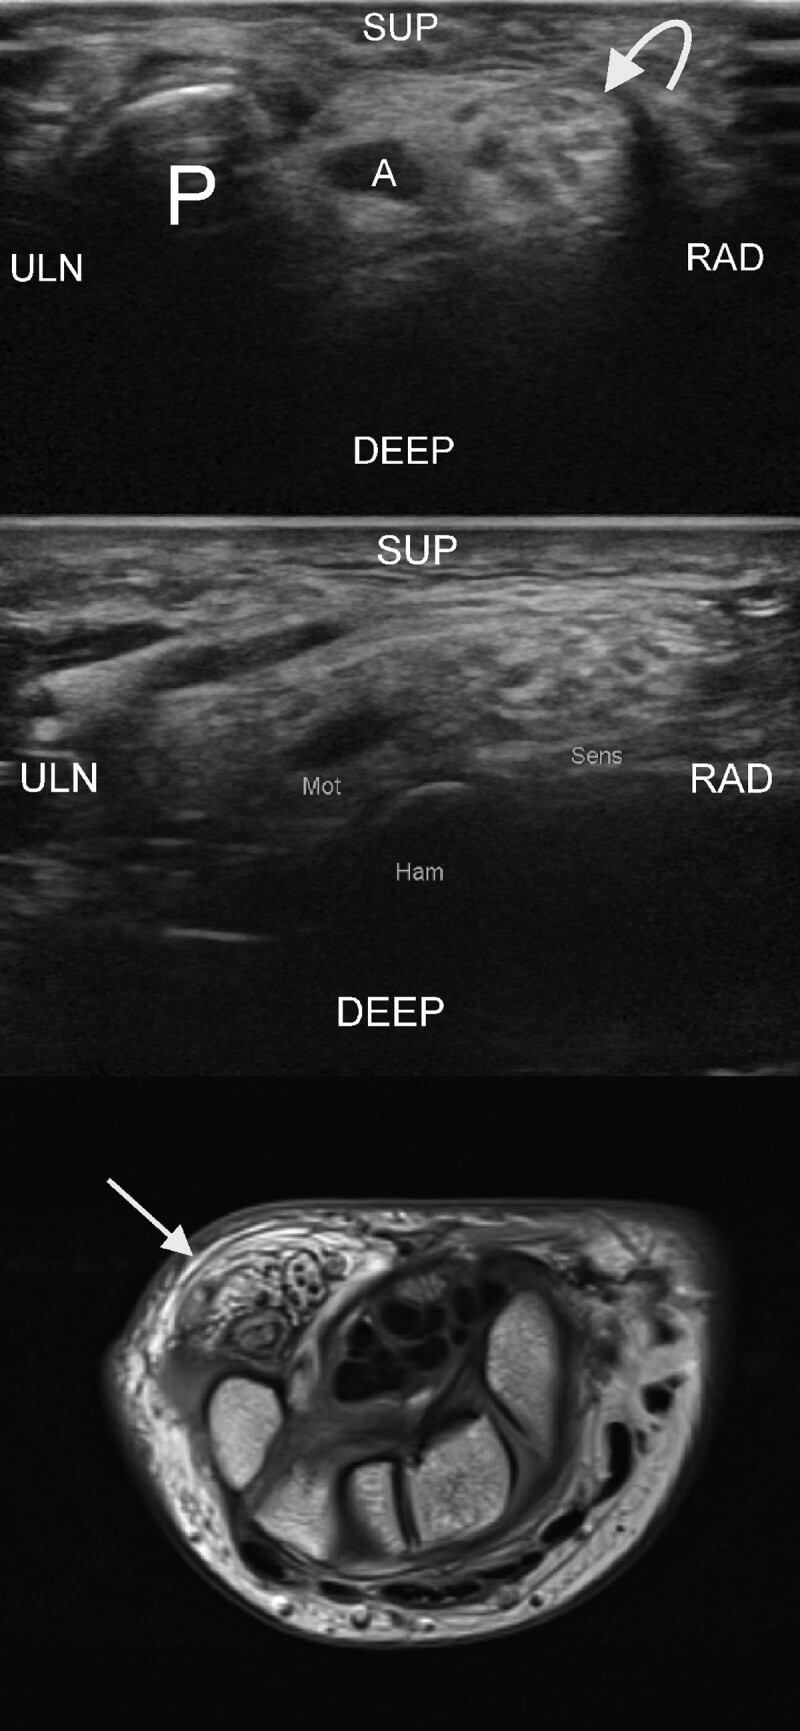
A. Patient 3 Top image. Transverse ultrasound image of the canal of Guyon in patient 3. Note pisiform bone (P), adjacent to it is the ulnar artery, and next to it the enlarged ulnar nerve. Middle image. Transverse ultrasound image at the level of the hook of the hamate. Note that the motor branch in the pisohamate hiatus appears relatively normal (it has been suggested motor nerves are less affected). The sensory branches are a bit flattened but the coaxial cable aspect is still obvious. Bottom image. MR imaging. Ulnar nerve in the canal of Guyon. Note markedly thickened nerve. Coaxial cable aspect is less obvious and appearance is more swirly-looking. (arrow)
